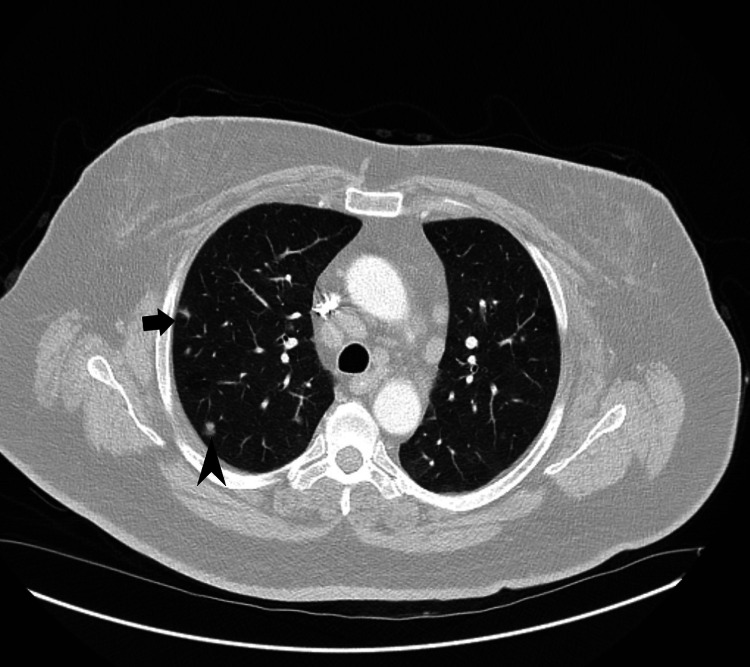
Transverse section of CT scan of the chest demonstrating multiple lung nodules in bilateral upper lobe distribution, including both cystic (block arrow) and solid (black arrowhead) lesions.
